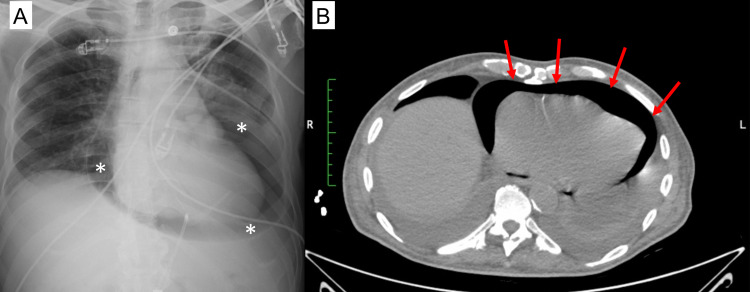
Post-Procedure Pneumopericardium Imaging (A) Portable chest X-ray with view of a large pneumopericardium (asterisks); (B) chest CT without contrast with cross-sectional view of pneumopericardium (arrows)
